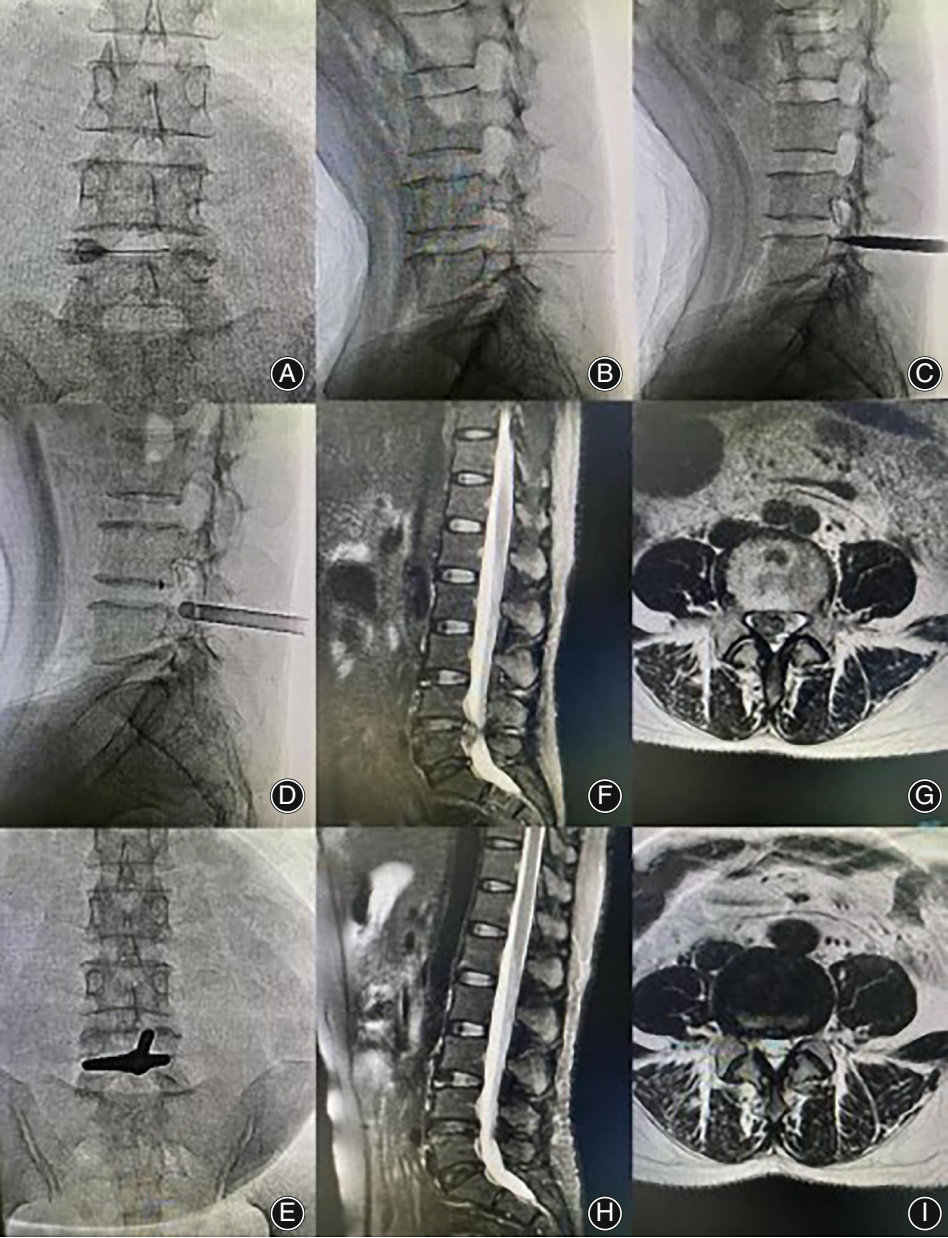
MPEID intraoperative fluoroscopy and preoperative and postoperative MRI. (A–E) Intraoperative radiographs, (F and G) Preoperative MRI, H and I: MRI 3 months after surgery.
